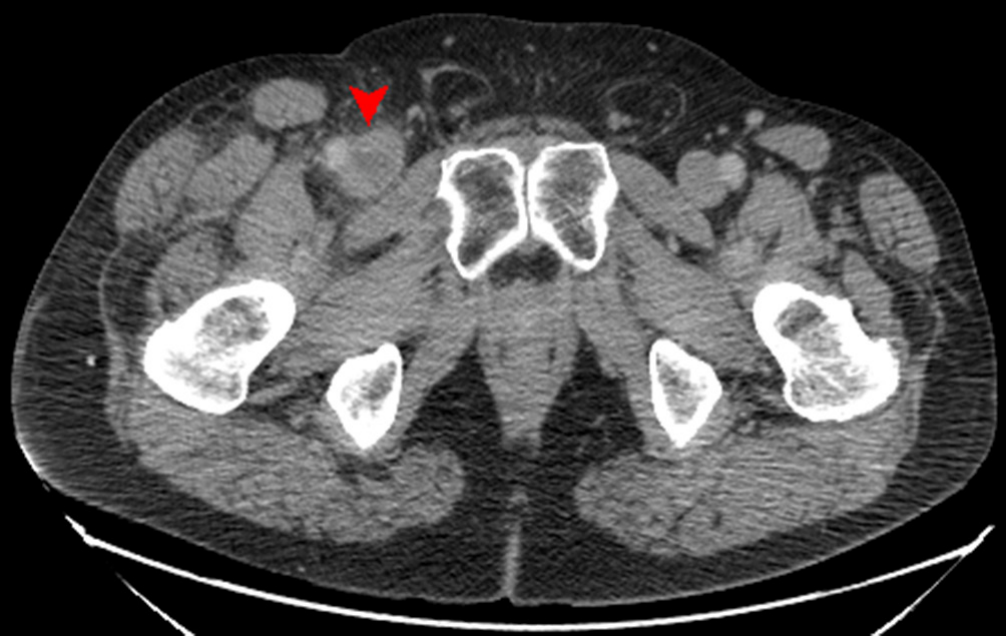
Axial CT scan showing a clot in the common femoral vein.
